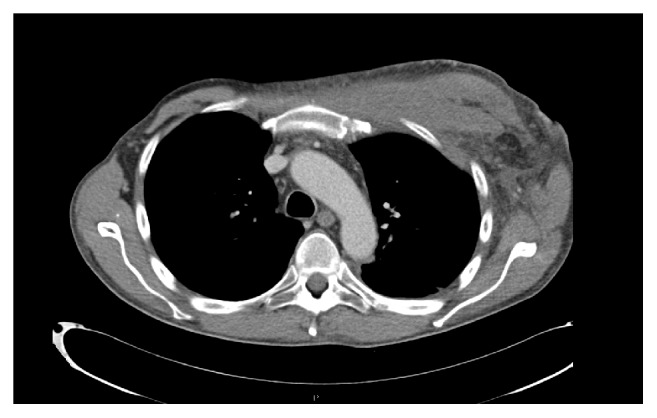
Thoracic Computed Tomography performed in the emergency department.
